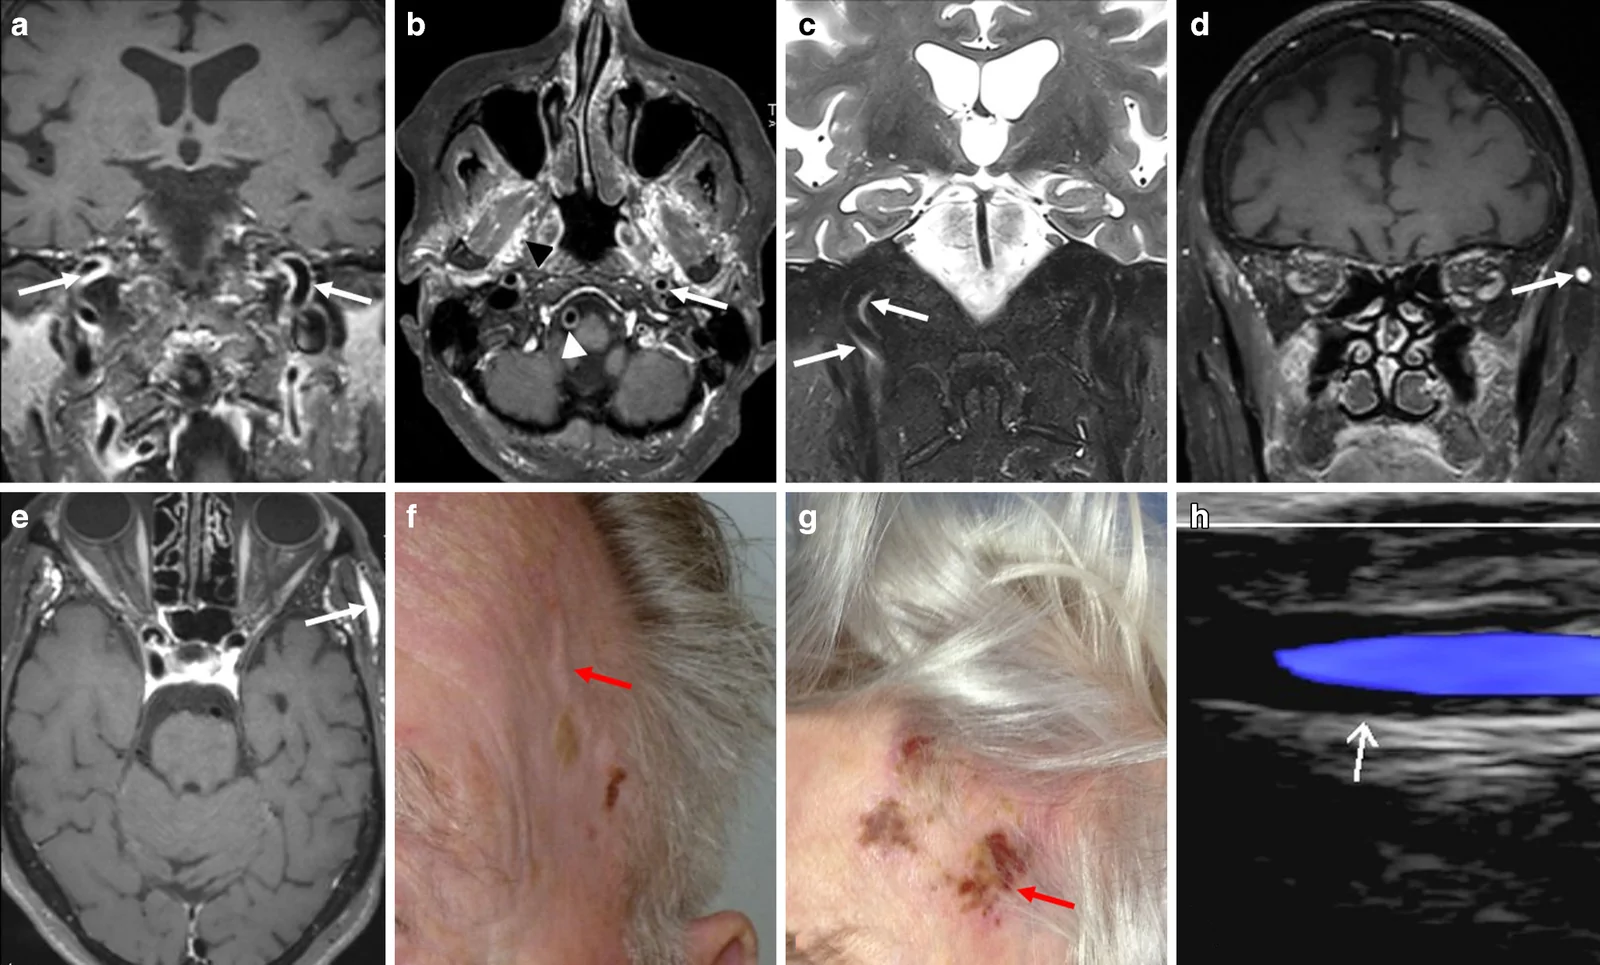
Giant cell arteritis (arteritis cranialis). a–e Vessel wall enhancement of the internal carotid artery (ICA; a, b arrow), the basilar artery (b white arrowhead) and the superficial temporal artery (d, e arrow) on post contrast (pc) T1 weighted images (WI); hyperintense signal on T2 WI (c arrow); note enhancement of the pterygoid muscles (b black arrowhead); f–h thickened superficial temporal artery (f arrow), cutaneous necrosis (g arrow) and “halo sign” in Doppler ultrasound (h arrow)
